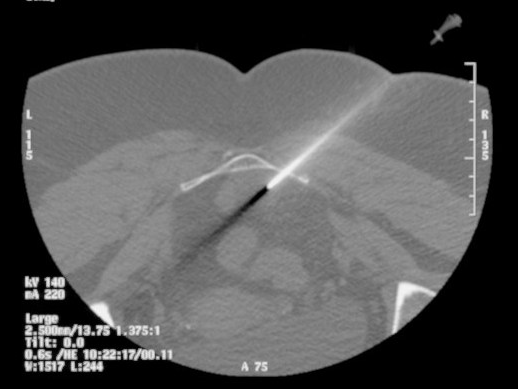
CT guided biopsy through presacral lesion using posterolateral approach.
